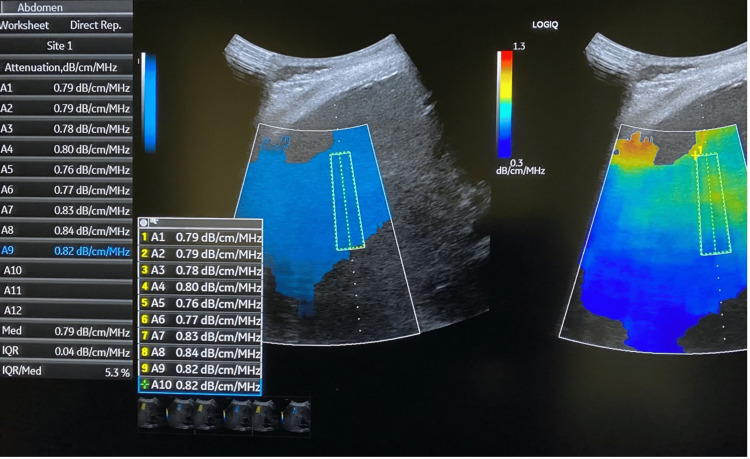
Ultrasound-guided attenuation parameter (UGAP) The ultrasound images shown are part of our team's original work and were obtained by the authors as part of this study.
